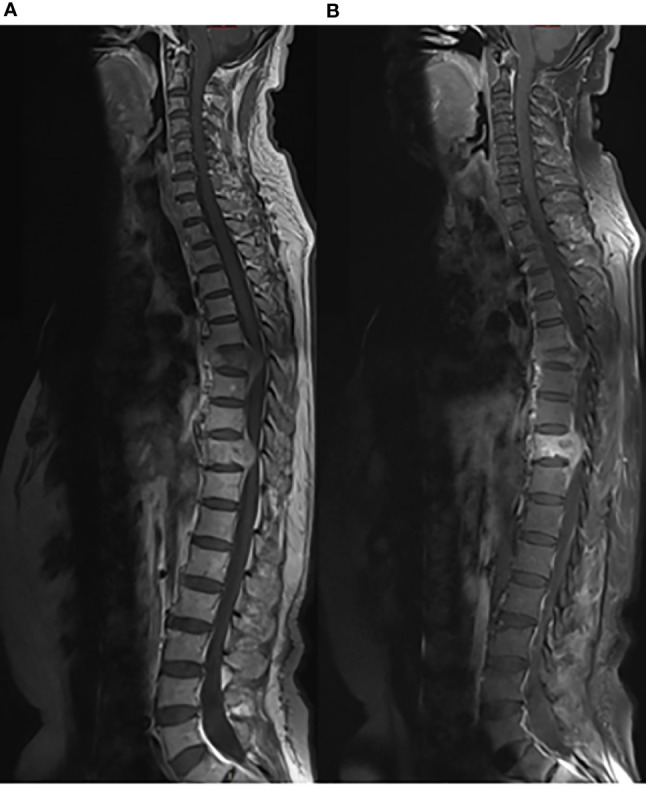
Whole spinal MRI findings on December 12, 2019. Sagittal planes of T1-weighted MRI of the whole spine showed metastases of the T7 and T10 vertebrae. (A) T1-weighted MRI. (B) Enhanced T1-weighted MRI.
